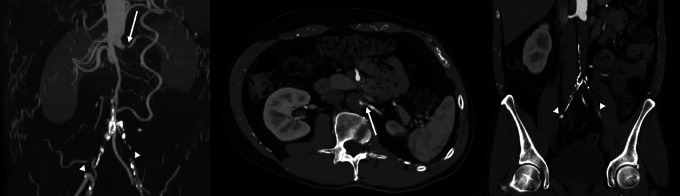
CT angiogram with virtual reconstruction showing aorto-iliac occlusion with ostial stenosis of the left renal artery (arrows) and re-injection distal into the common iliac arteries (arrowheads).
